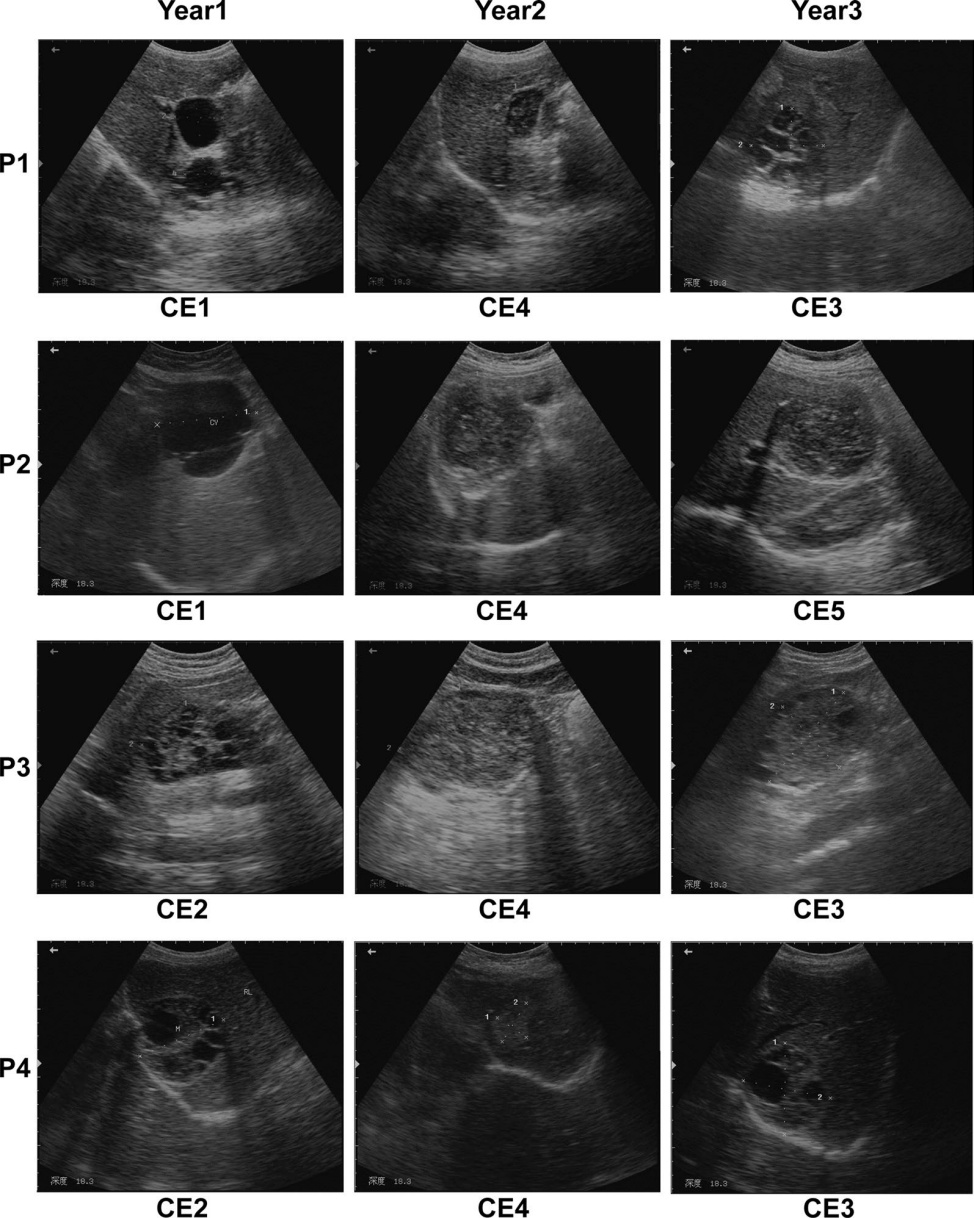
Ultrasound images of four CE patients (P1-P4) followed-up for three consecutive years
